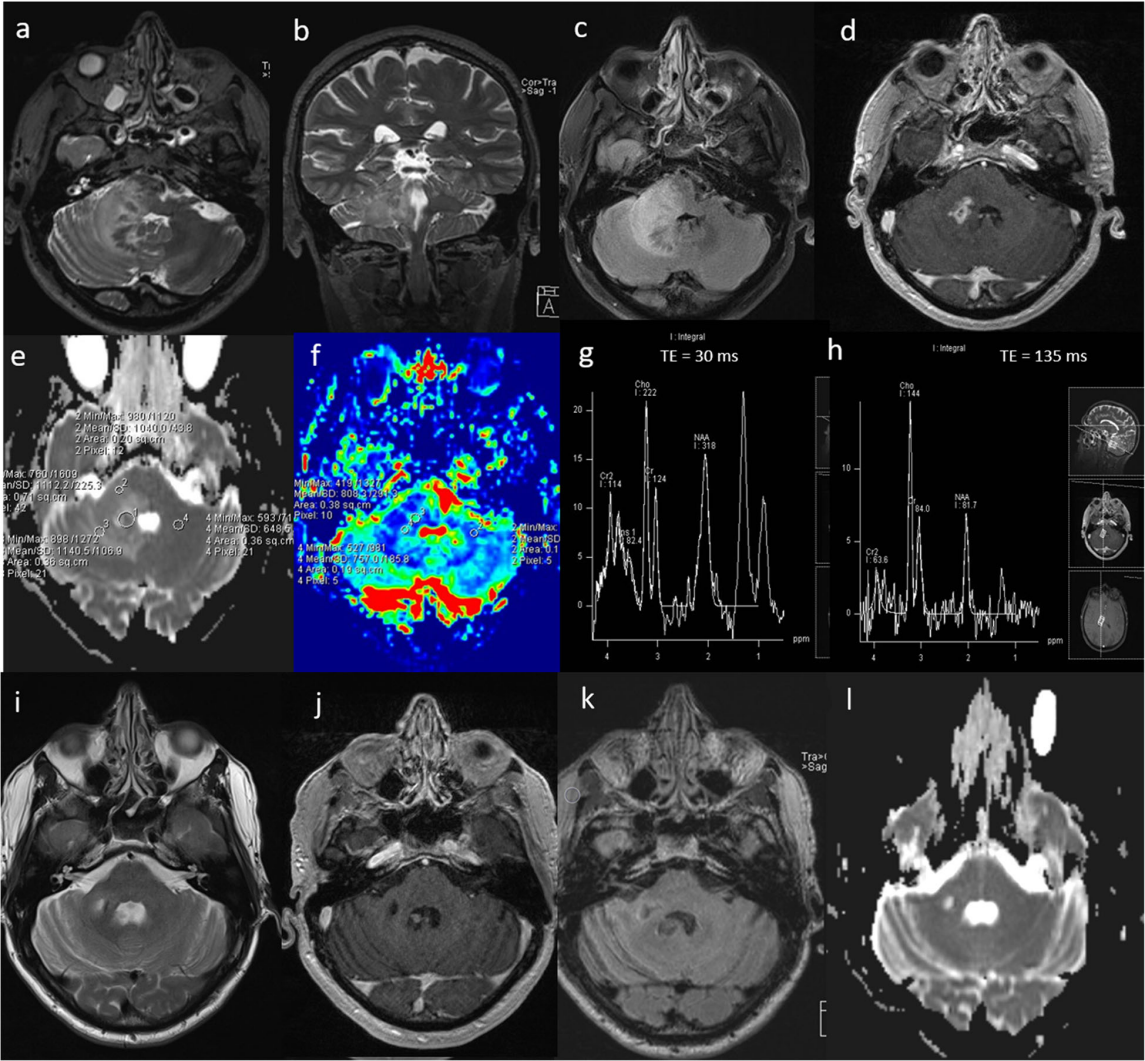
Neurosarcoidosis. Known case of systemic sarcoidosis. Conventional MRI Findings: a, b Axial and coronal T2W, (c) axial FLAIR and (d) post-contrast T1W sequences, showing a diffuse infiltrative lesion with enhancing foci in the right cerebellar peduncle extending to the brainstem, mimicking tumour. Multiparametric MRI: e DWI shows focal areas of low ADC. f PWI shows low perfusion in comparison to the contralateral side. g MRS with a short TE (30 ms) shows moderately high Cho/Cr ratio (< 2), near normal NAA/Cr and mI/Cr, presence of glutamate and glutamine at 2.4–2.6 ppm and large lipid peaks at 0.9 and 1.3 ppm suggesting necrosis. h MRS with a TE 135 ms shows slightly low NAA/creatine ratio and absence of lactate. In this case, the findings of low perfusion (< 2), absence of a lactate peak and presence of glutamine and glutamate favour an inflammatory aetiology such as neurosarcoidosis rather than a high-grade glioma. A tapering dose of oral prednisolone was commenced, during which neurological symptoms improved. Three-month follow-up MRI; (i) axial T2W, (j) post-contrast T1W, (k) FLAIR and (l) ADC sequences show near complete resolution of the lesion after treatment with steroids
